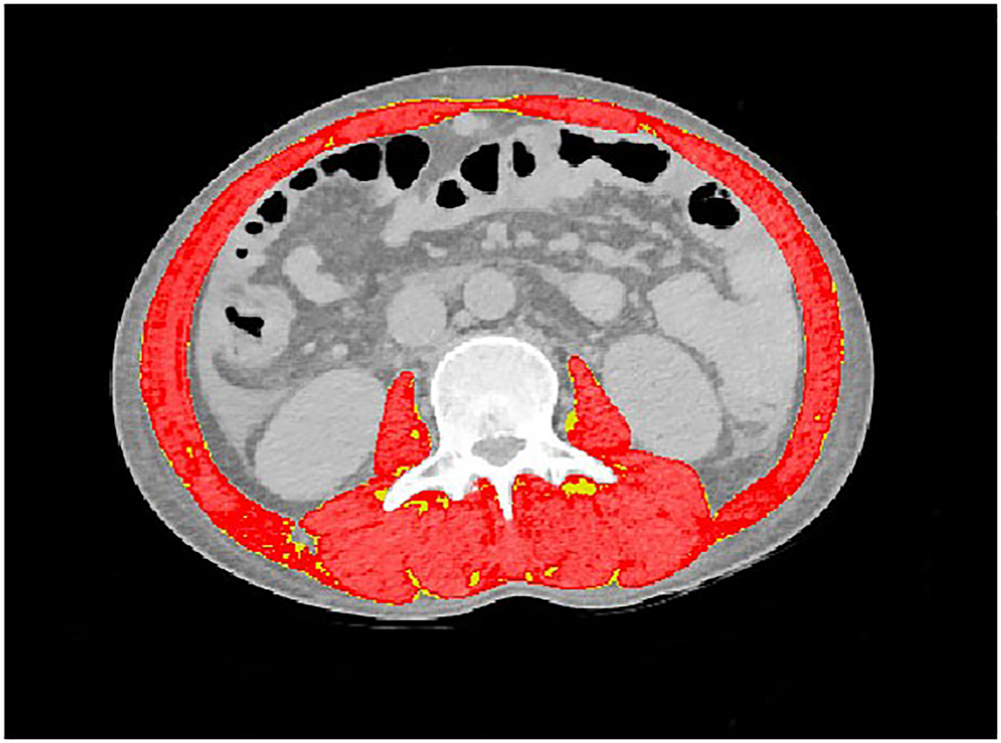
A representative computed tomography image at the L3 vertebral level for the quantification of skeletal muscle area (red) and intermuscular adipose tissue (yellow).
